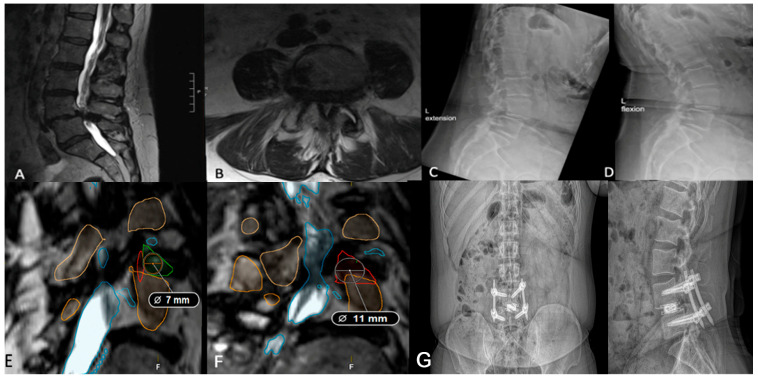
(A,B) Preoperative lumbar MRI of a patient with grade II L4/5 spondylolisthesis and severe low back pain refractory to conservative management. (C,D) Preoperative flexion and extension radiographs assessing segmental instability. (E) Axial segmentation demonstrating a 7 mm trans-Kambin working corridor. (F) Ipsilateral corridor measuring 11 mm, suggesting the TF-TLIF may be appropriate. (G) Postoperative standing lateral and anteroposterior radiographs demonstrating appropriate implant placement following TF-TLIF.
